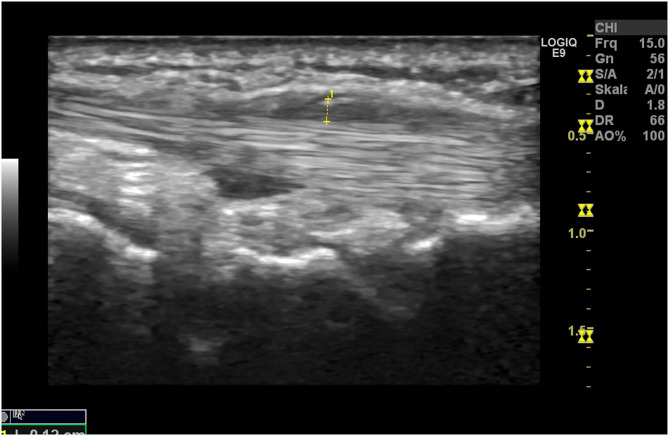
Ultrasound assessment of flexor tendinitis.
